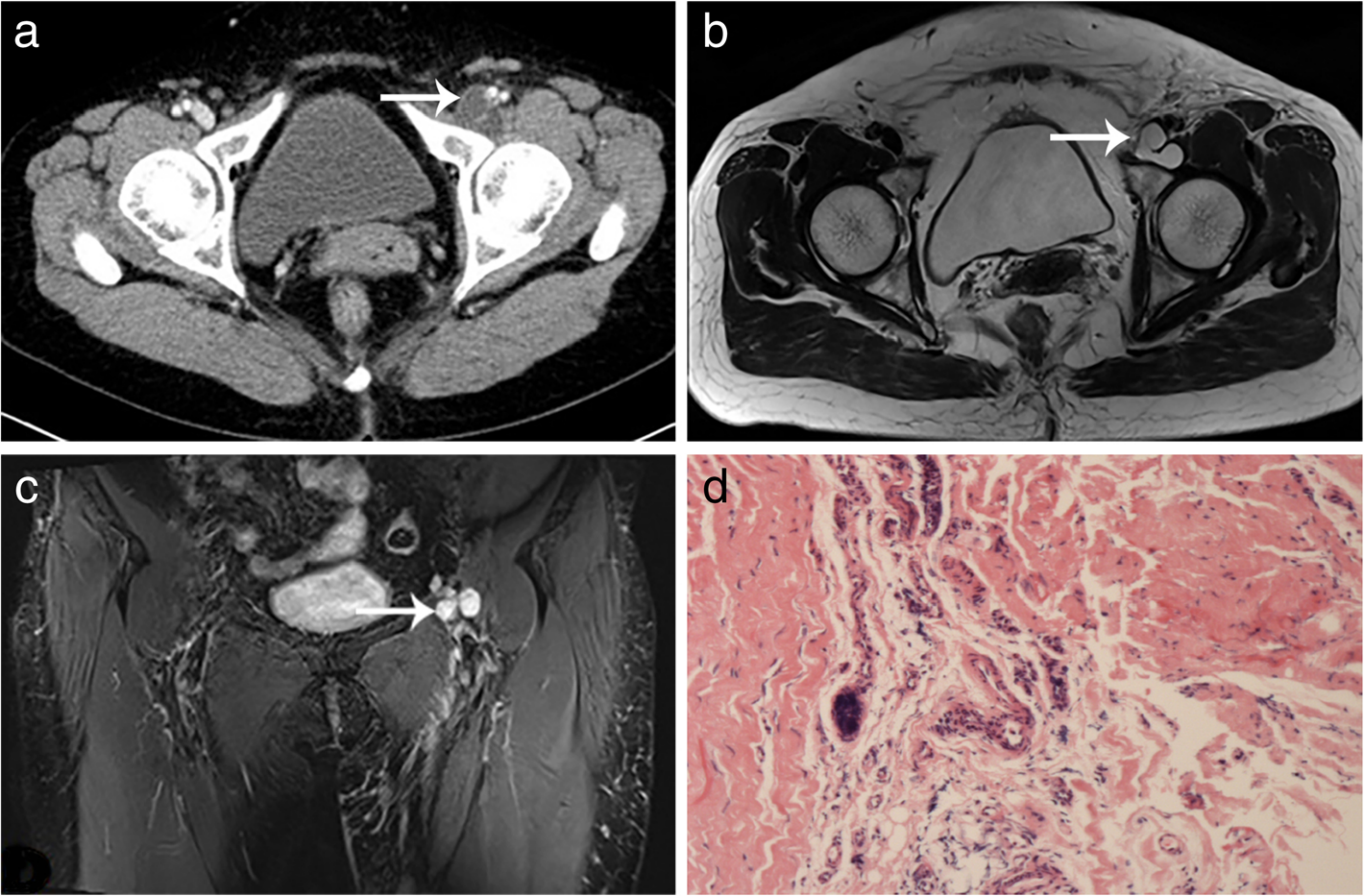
Image data and pathology result for patient NO.2. a. A CT and b. and c. MRI showed a cystic space-occupying lesion (white arrow) in the inguinal region, leading to severe stenosis of the common femoral vein. d. The pathology result confirmed a synovial cyst
